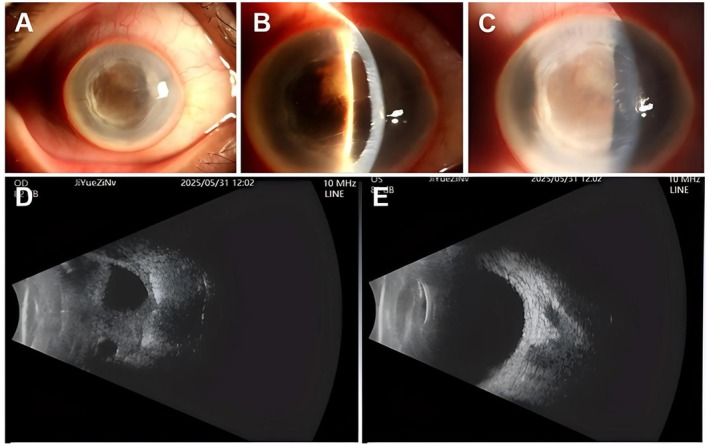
Anterior segment photography and B‐scan ultrasonography at 6‐month follow‐up. (A) The right eye showed conjunctival hyperemia. (B) The cornea was thickened with a central anterior chamber depth of 2 CT. (C) Extensive peripheral anterior synechiae and iris neovascularization (rubeosis iridis) with concomitant hemorrhage. (D) The right eye showed disorganized intraocular structures and a shortened axial length, consistent with phthisis bulbi. (E) The left eye showed persistent mild vitreous opacity.
